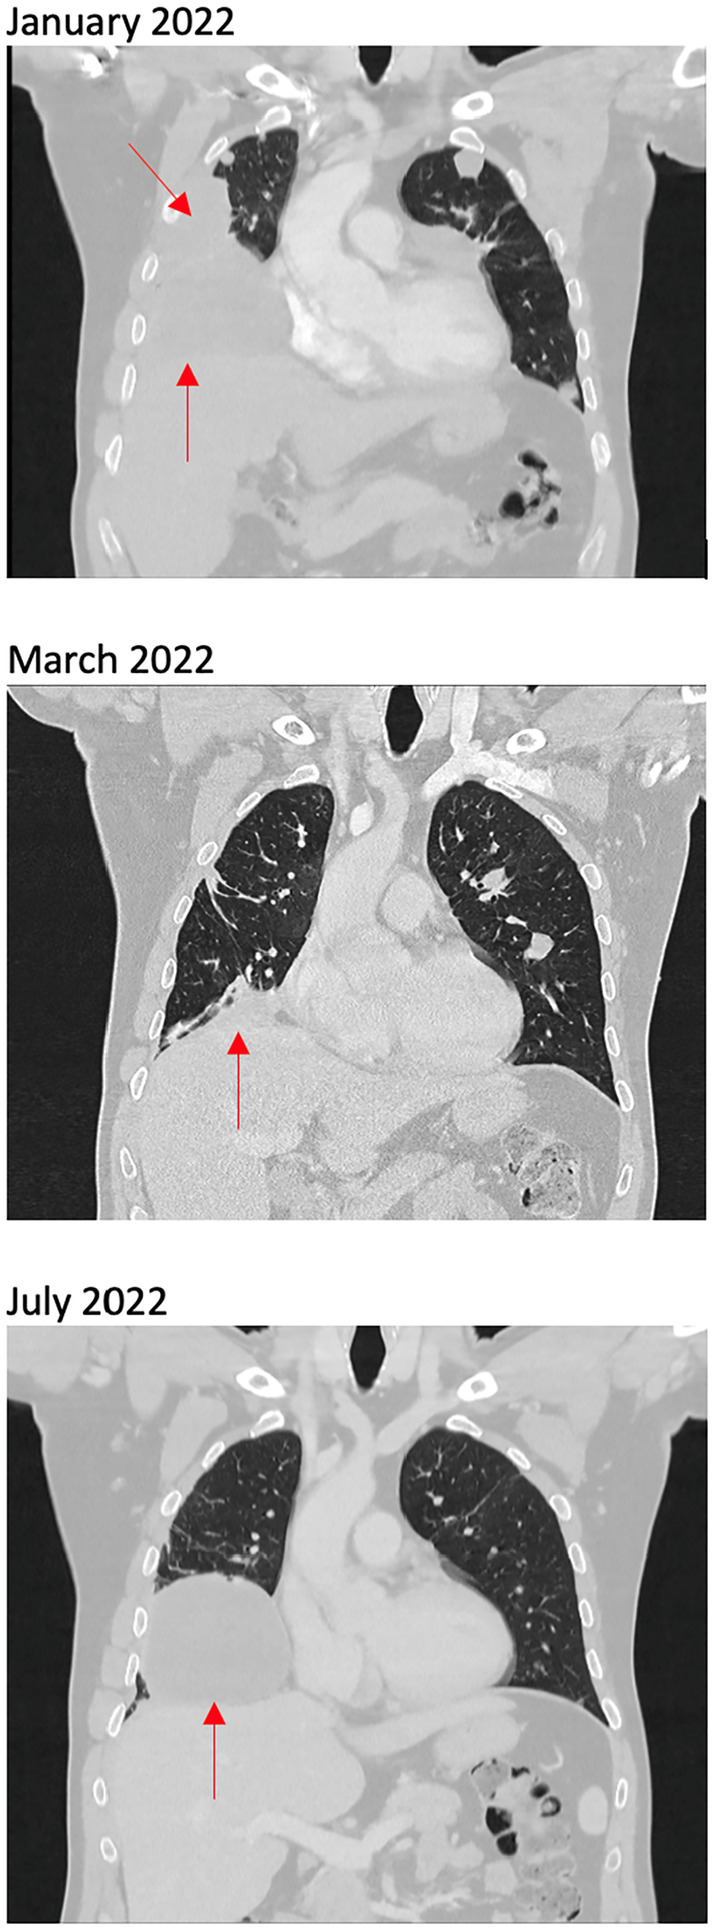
CT scan at baseline, during response to alectinib, and at progression.
